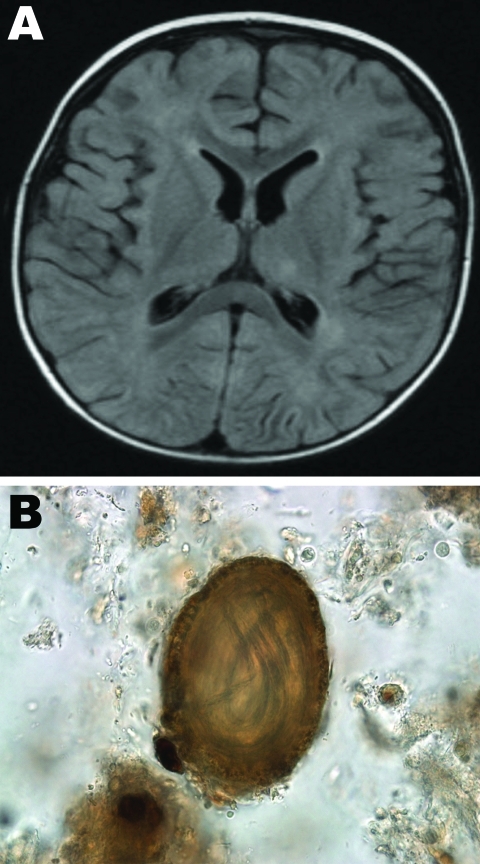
A) Magnetic resonance imaging of the brain of a 14-month-old child with baylisascariasis encephalitis. B) *Baylisascarasis procyonis* embryonated egg found in wet preparation of raccoon feces; original magnifi cation ×100.
